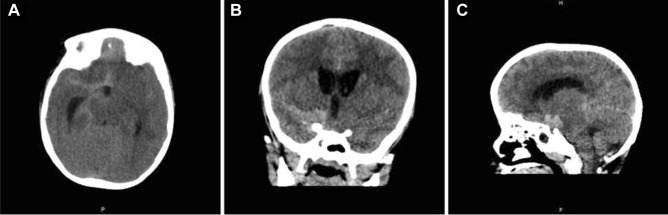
This is the initial computerized tomography (CT) scan with axial, coronal, and sagittal views demonstrating the basal subarachnoid hemorrhage (A–C)
